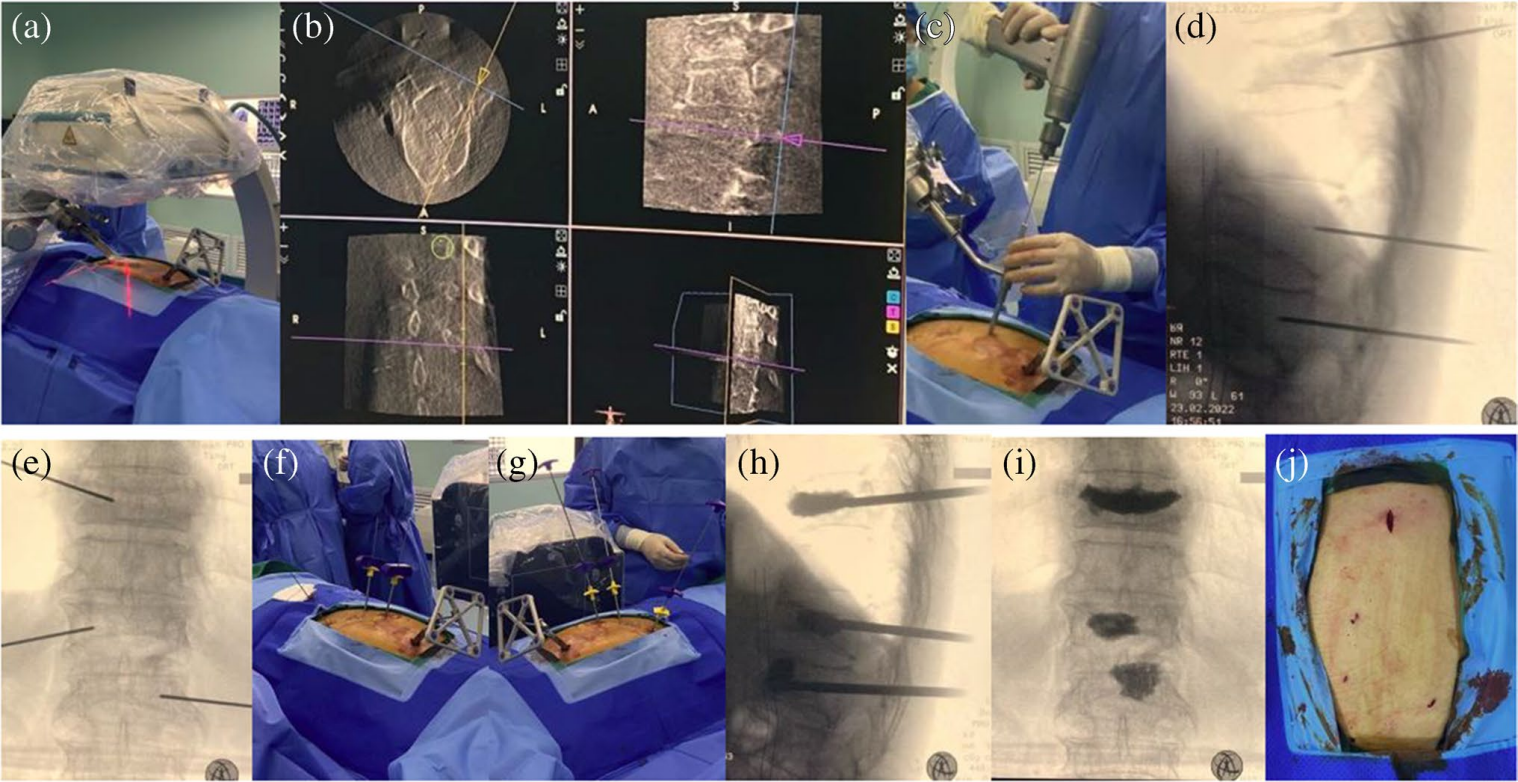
The TiRobot-assisted procedure in a 73-year-old female patient with T10-L1 vertebral compression fracture: (a) Robot registration and scanning intra-operative imaging; (b) trajectory planning in robot workstation; (c) inserting the guidewire with robot-assisted; (d), (e) intra-operative X-ray film; (f) inserting the sleeve; (g) cement injection; (h), (i) intra-operative X-ray film; (j) incision
